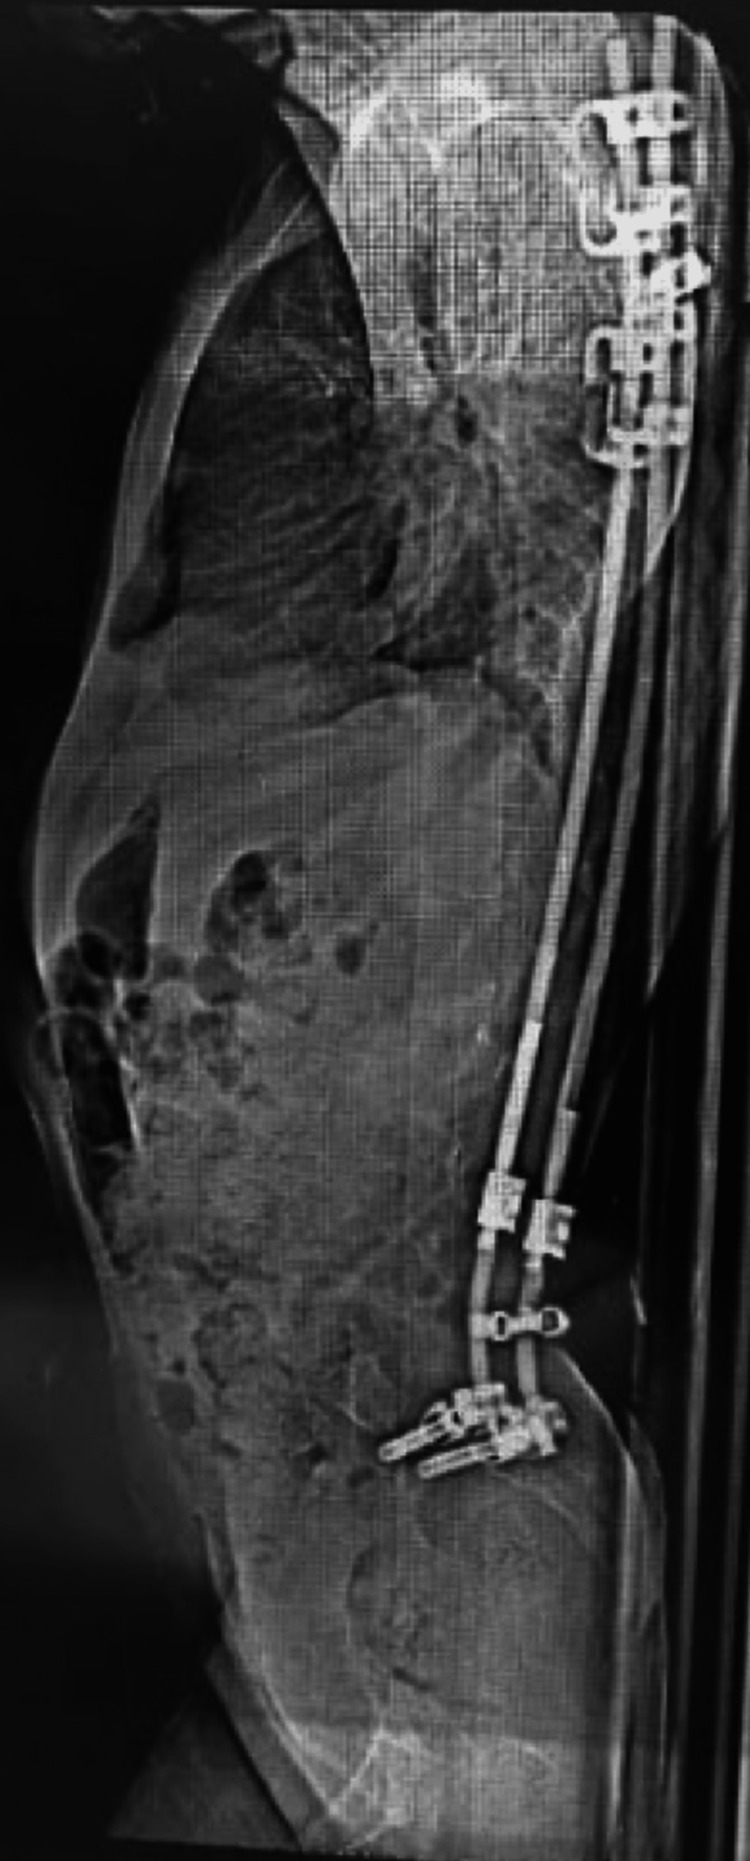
Latest follow-up lateral spine radiograph showing the proper positioning of the whole construct
